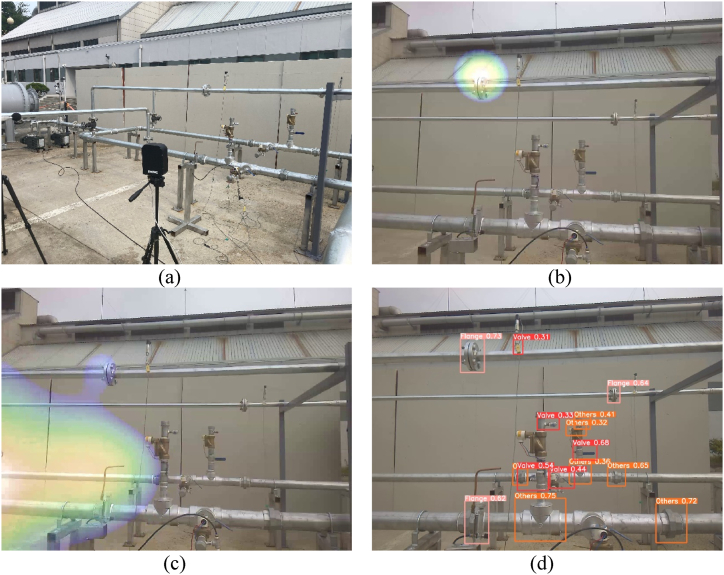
(a) Experiment set-up for case #5. (b) The ultrasonic camera detects the leaky flange without noise. (c) The ultrasonic camera output showing that the sound reflected by the safety wall works as a noise source. (d) Pipe joint detection result from the image taken by the ultrasonic camera.
